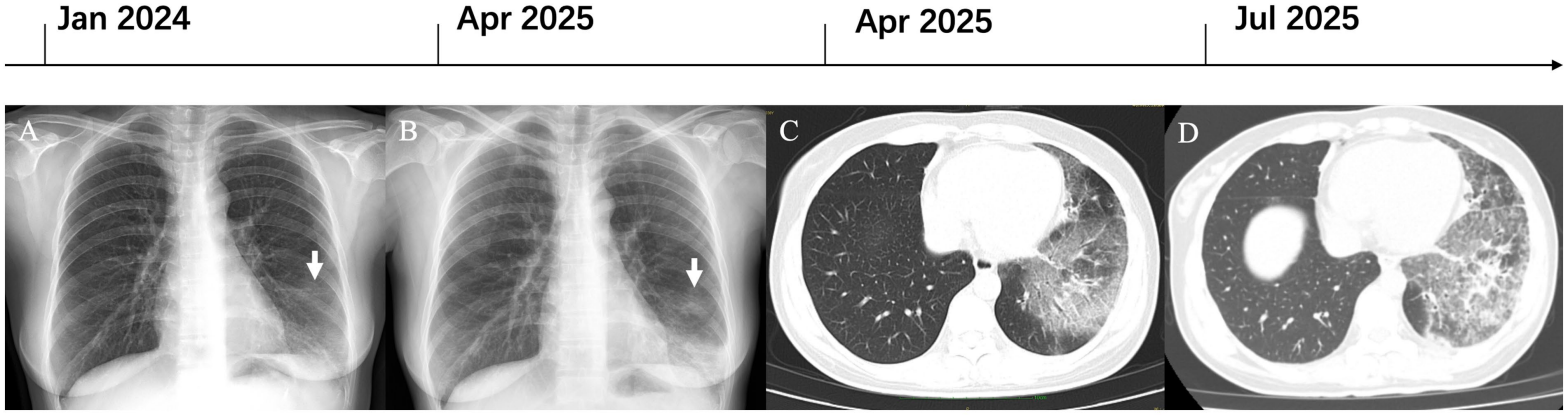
Imaging reveals progressive lung opacities in pulmonary MALT lymphoma. (A) Patchy opacification confined to the left lower lobe (white arrow, Jan 2024). (B) Progression to consolidation with patchy infiltrates (white arrow, Apr 2025). (C) Multifocal flocculent opacities with bronchovascular markings on the contrast CT scan, predominantly in the left lower lobe. (D) Persistent multifocal patchy and flocculent opacities, with new subtle interlobular septal thickening (July 2025). MALT, mucosa-associated lymphoid tissue.
